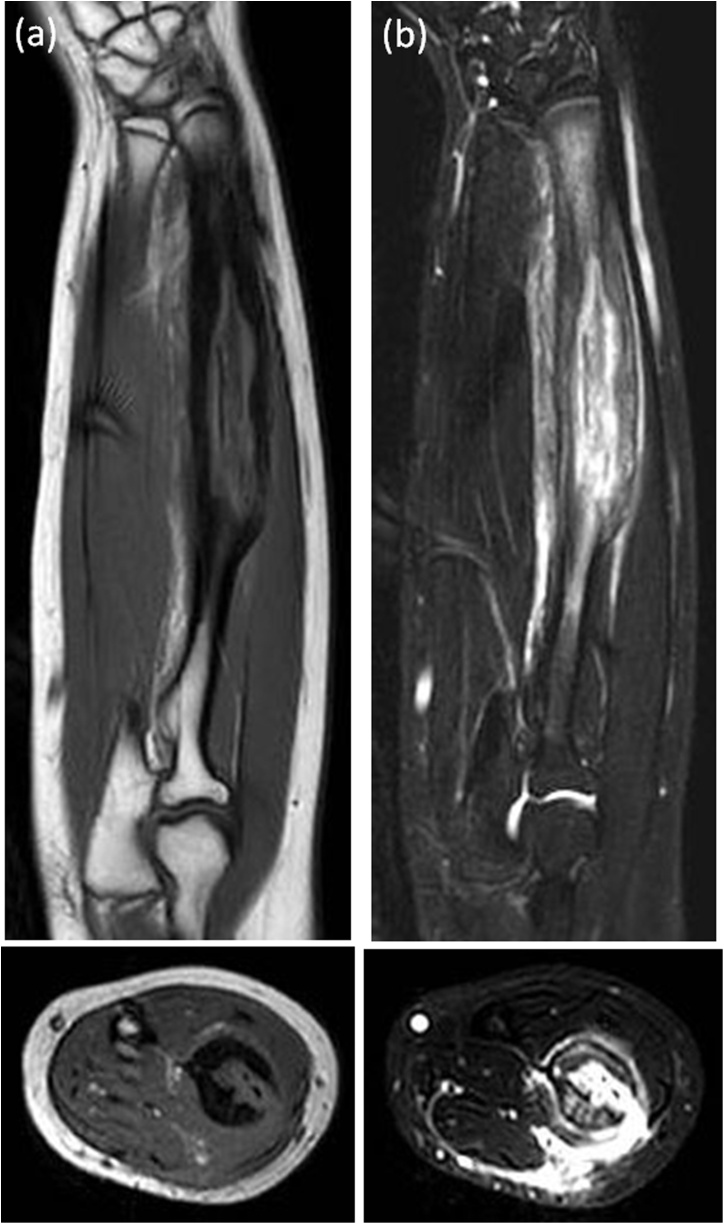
MRI. (a), (b) An MRI shows that the lesion is hypointense on T1-weighted imaging and hyperintense on T2-weighted imaging inside the radius. (c), (d) Extraosseous abscess is well visualized as a hypointense lesion on hyperintense lesion on T2WI outside the radius.
